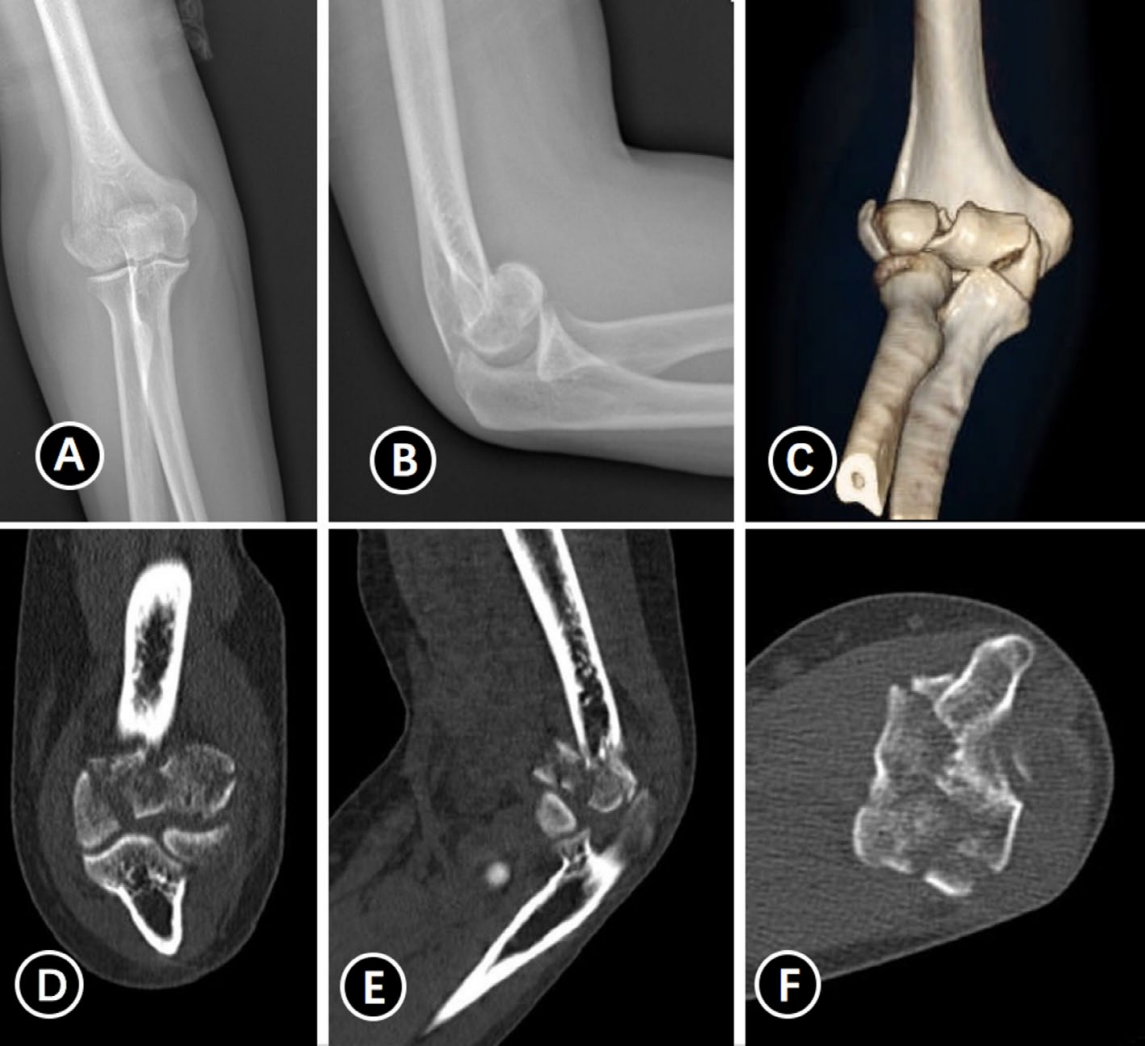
Preoperative radiography and CT examination and three‐dimensional construction of a 54‐year‐old female with Duckerley type IIIB distal humerus fractures (A–F).
